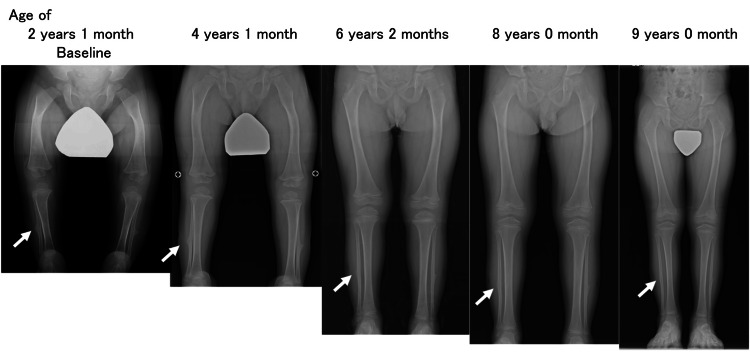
Changes in lower extremity radiographic findings after initiating asfotase alfa Improvement in femoral deformity, bow legs, and bone spur of the fibula (arrow).
